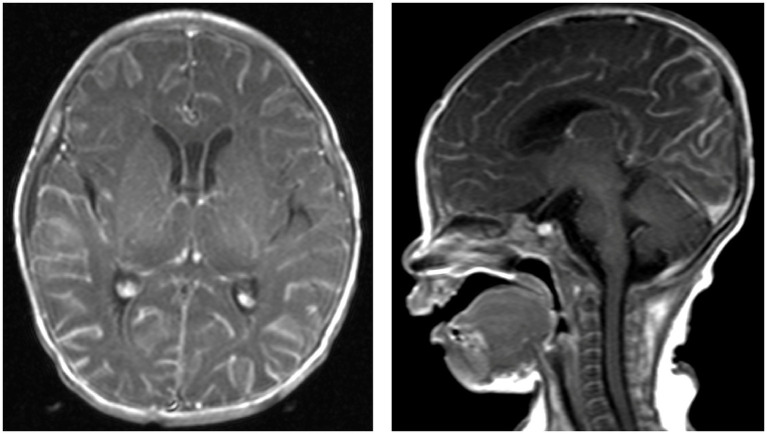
Cranial MRI plain and enhanced scans: Heterogeneous signal intensity of the brain parenchyma in both cerebral hemispheres, with diffuse, pronounced thickening and enhancement of the bilateral meninges.
